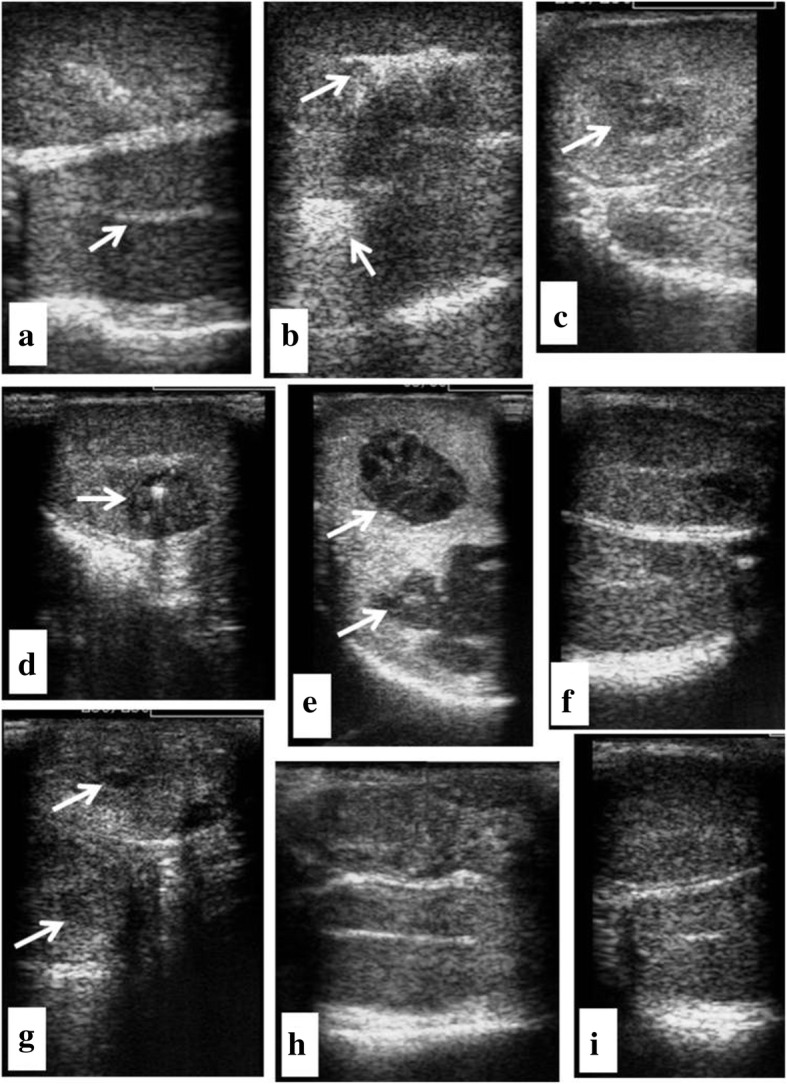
Ultrasonographic view of testes before (a) and 0 (b), 3, (c), 6 (d), 12 (e), 21 (f), 28 (g), 35 (h) and 37 (i) days after injection of zinc gluconate. a before injection, arrow indicates rete testis; b hyperechogenic echotexture indicates injected zinc gluconate solution (arrow); c, d, e, g arrows indicate formation and elimination of cystic like structures; h, i ultrasound view of the testes at the end of the study (day 37)
